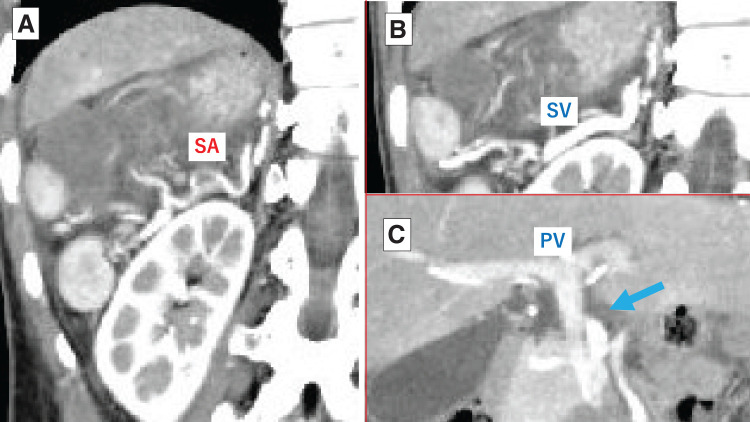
Contrast-enhanced CT on admission. (A) The SA branches into multiple vessels, forming an arterial arcade in the periphery that supplies each spleen. (B) The SV runs along the right dorsal side, anterior to the SA. (C) The SV joins from the left to form the PV (blue arrow). PV, portal vein; SA, splenic artery; SV, splenic vein
